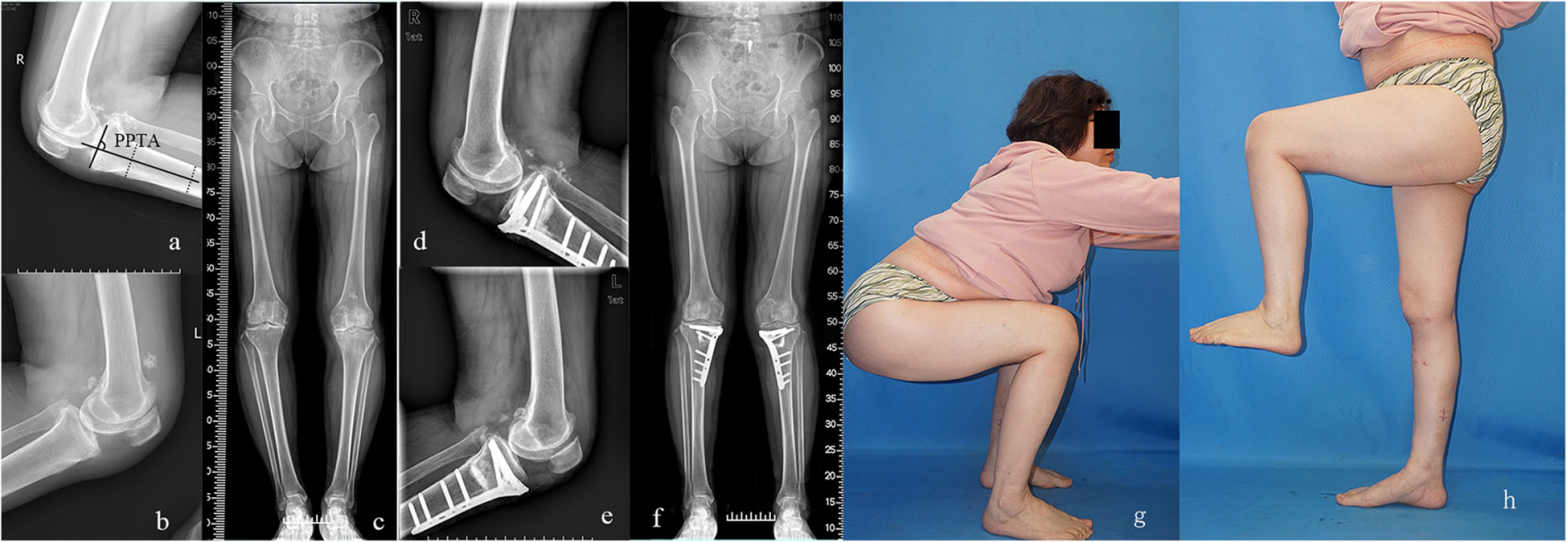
A 58-year-old female underwent tibial condylar valgus osteotomy (TCVO). Pre-operative lateral radiographs of the right (a) and the left (b) knee joints. PPTA was defined as posterior proximal tibial angle (a). Pre-operative standing radiograph of the lower extremities (c). Post-operative lateral radiographs of the right (d) and the left (e) knee joints at 2 years after surgery. Post-operative standing radiograph of the lower extremities at 2 years after surgery (f). She was able to jog and squat after TCVO
